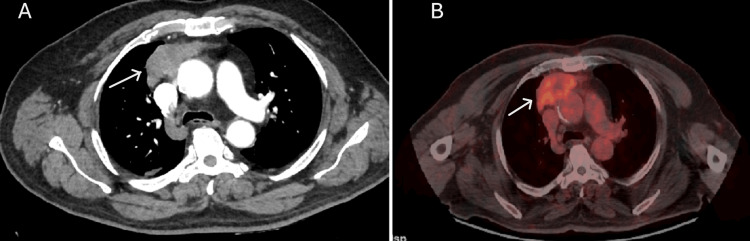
CT and PET/CT images of the anterior mediastinal mass CT (A) and PET/CT (B) images show a lobulated, irregularly marginated anterior mediastinal mass measuring 7.5 × 2.9 × 4.6 cm. The mass is inseparable from the pericardium and demonstrates focal obliteration of fat planes adjacent to the ascending aorta (white arrow). PET/CT shows increased F-18 fluorodeoxyglucose uptake (SUVmax: 5.91), indicating glucose metabolism in the tumor.
